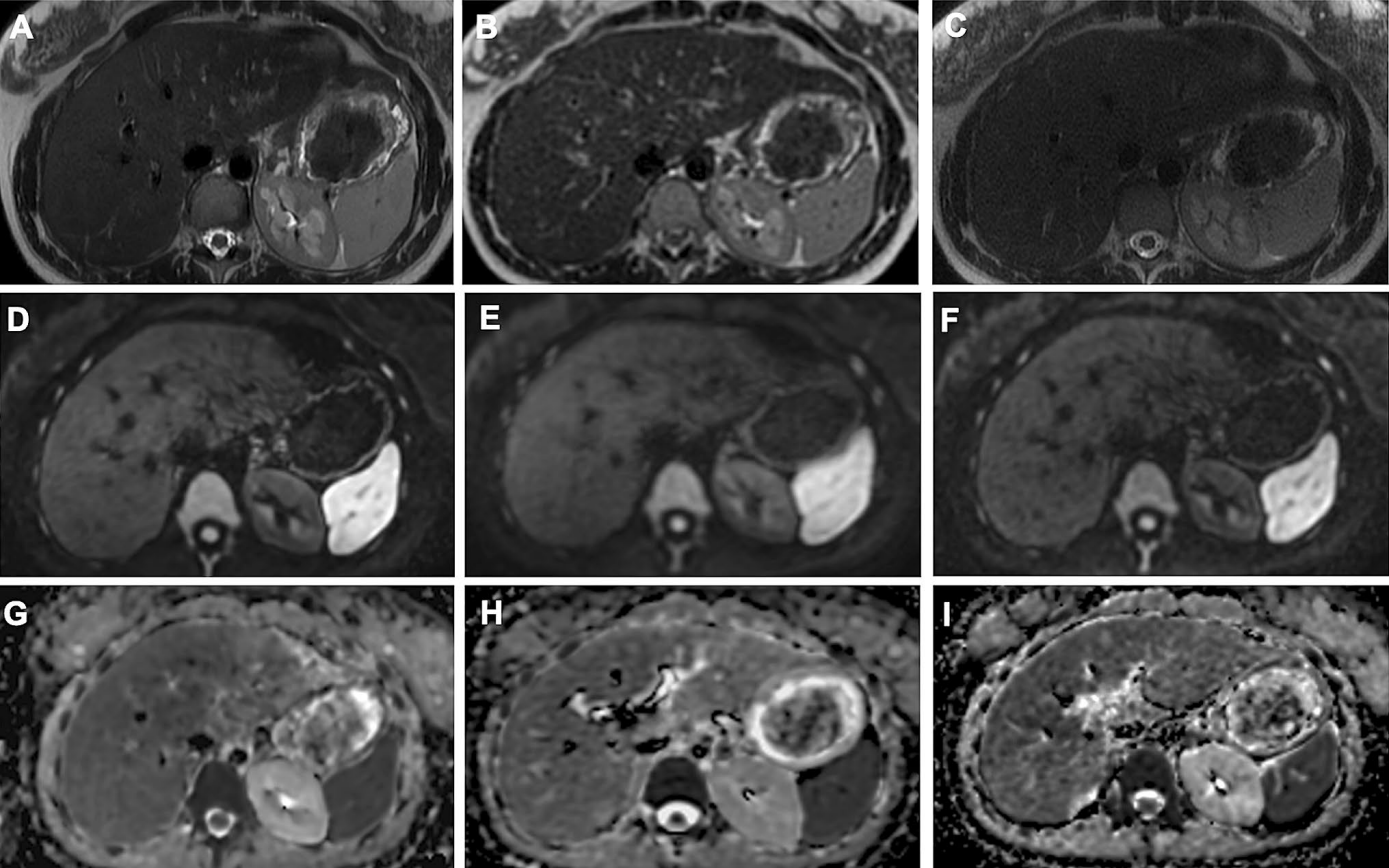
Comparison of ARDL, NAÏVE, NON-DL dataset on SSFSE T2, DWI and ADC. Female, 28-years old underwent unenhanced upper-abdomen MRI. Axial images showing SSFSE T2 sequences with ARDL a, NAÏVE b, NON-DL c dataset respectively; DWI sequences with ARDL d, NAÏVE e, NON-DL f; ADC maps with ARDL g, NAÏVE h and NON-DL i. Anonymized datasets were obtained to perform qualitative image analysis with 5-point Likert scale assessing Sharpness, Contrast, Truncation artefacts, Motion artifacts and Overall image quality. ARDL dataset showed higher image quality in all datasets in terms of overall image quality compared to NAÏVE and NON-DL dataset.
